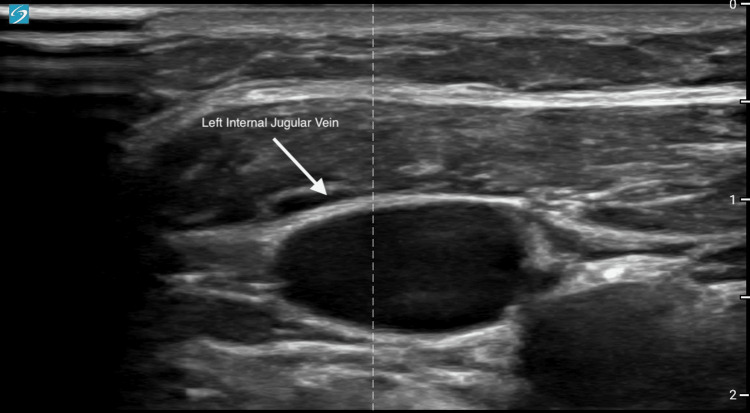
Cross-sectional ultrasound image of the left internal jugular vein
